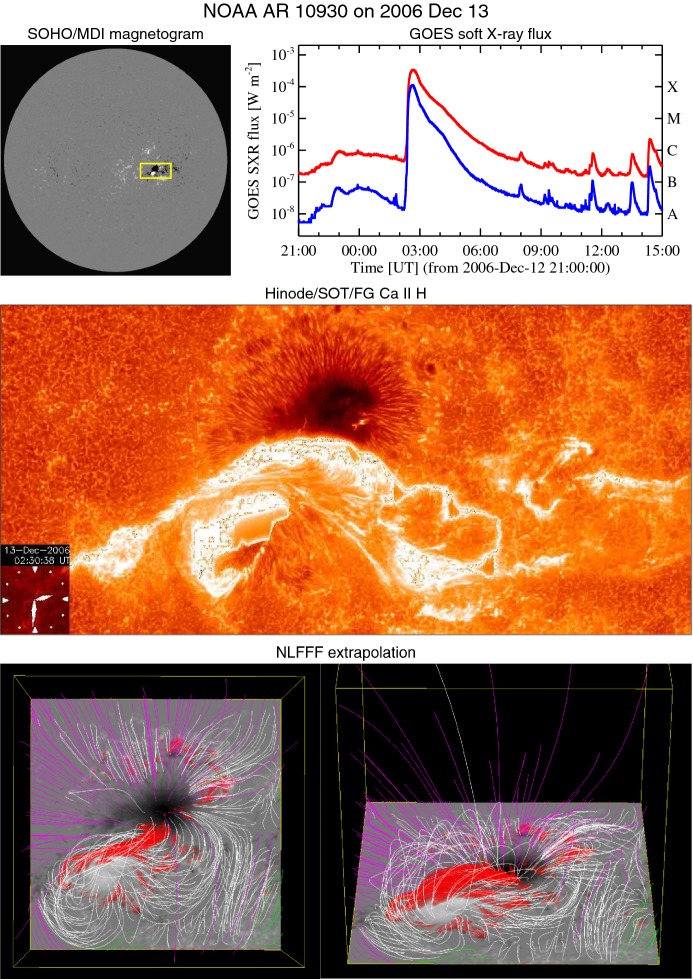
X3.4-class flare in AR NOAA 10930. The panels show full-disk magnetogram from Michelson Doppler Imager (MDI) aboard the Solar and Heliospheric Observatory (SOHO), GOES soft X-ray light curves for 1–8 Å (red) and 0.5–4.0 Å (blue), and Hinode/SOT/FG Ca ii H image (see also the accompanying movie), whose FOV is indicated by a yellow box in the magnetogram. Hinode image courtesy of Joten Okamoto (ISAS/JAXA and NAOJ). The bottom panel displays the computationally extrapolated magnetic field lines before the X3.4 flare using the NLFFF method. The red isosurface shows where the electric current is highest. Image reproduced by permission from Schrijver et al. (2008), copyright by AAS
